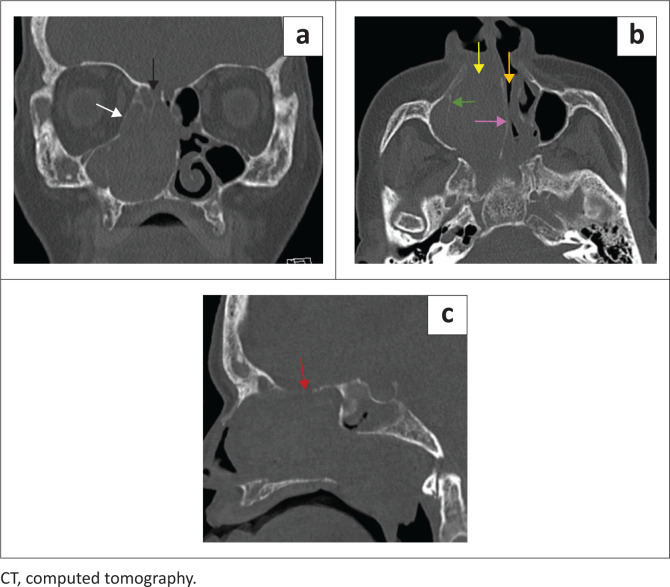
Bony window coronal (a), axial (b) and sagittal (c) CT images demonstrating displacement, remodelling and dehiscence of the right cribriform plate (black arrow), planum sphenoidale (red arrow), lamina papyracea (white arrow), medial maxillary sinus wall (green arrow) and nasal septum (purple arrow). Soft tissue mass obstructing the right nasal passage (yellow arrow). Displacement of the bony nasal septum to the left, obstructing the left nasal passage (orange arrow).
